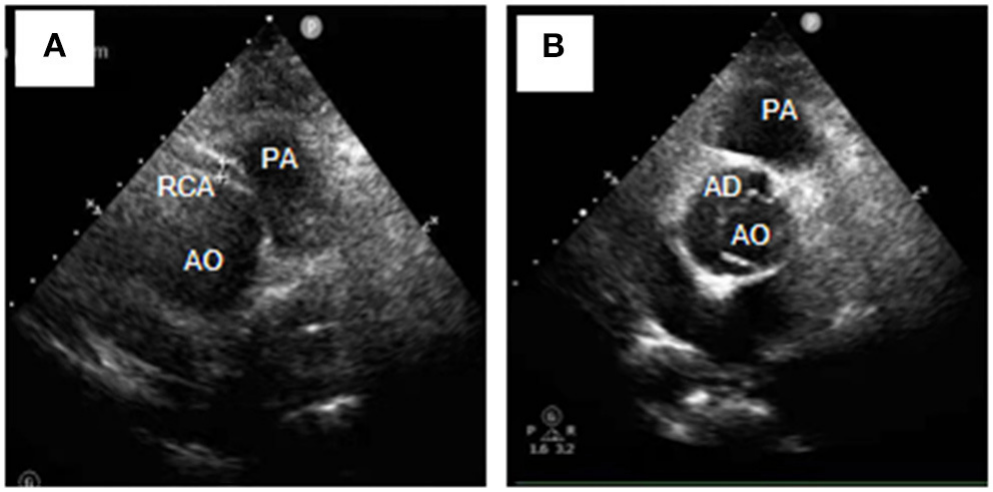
Transthoracic echocardiography (Short-axis view at the great artery) revealed Stanford type A aortic dissection (A), avulsion of the RCA, and the RCA originating from the left coronary sinus (B). RCA, right coronary artery; AD, aortic dissection; AO, aortic; PA, pulmonary artery.
